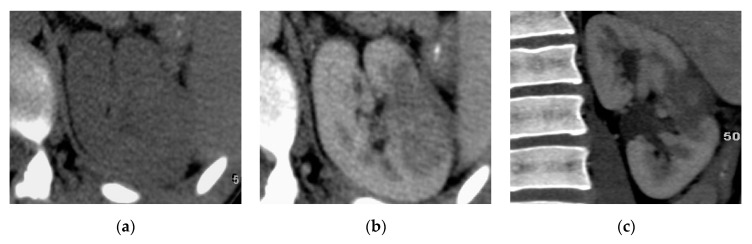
Left acute pyelonephritis. (a) Axial non-contrast CT image shows a slight focal contour bulge at the middle of the kidney. (b) Axial and (c) coronal reformatted post-contrast CT images show mildly enhancing subcapsular lesion of relative wedge shape extending to the medulla with thickened related perinephric fascia with distorted perinephric fat.
